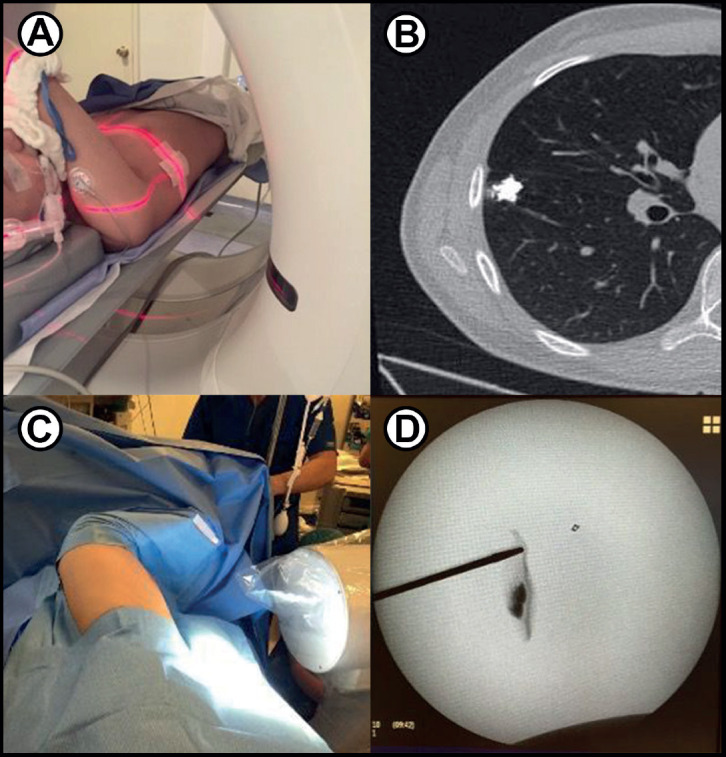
(a): Preoperative marking. A paediatric patient with osteosarcoma and a small right-sided lung nodule is marked under CT guidance. Just before surgery, interventional radiology injects 0.1 mL of lipiodol into the nodule to allow fluoroscopic visualisation during thoracoscopy. (b): Post marking CT image of the patient demonstrates the contrast-enhanced nodule. (c): During surgery, fluoroscopy aids in localising the lung nodule for full thoracoscopic resection. (d): Intraoperative fluoroscopy image identifies the small, intra-parenchymal nodule. (a–d) are courtesy of Gloria Gonzalez, MD. Paediatric Transplant and Oncology Surgeon, Cleveland Clinic.
